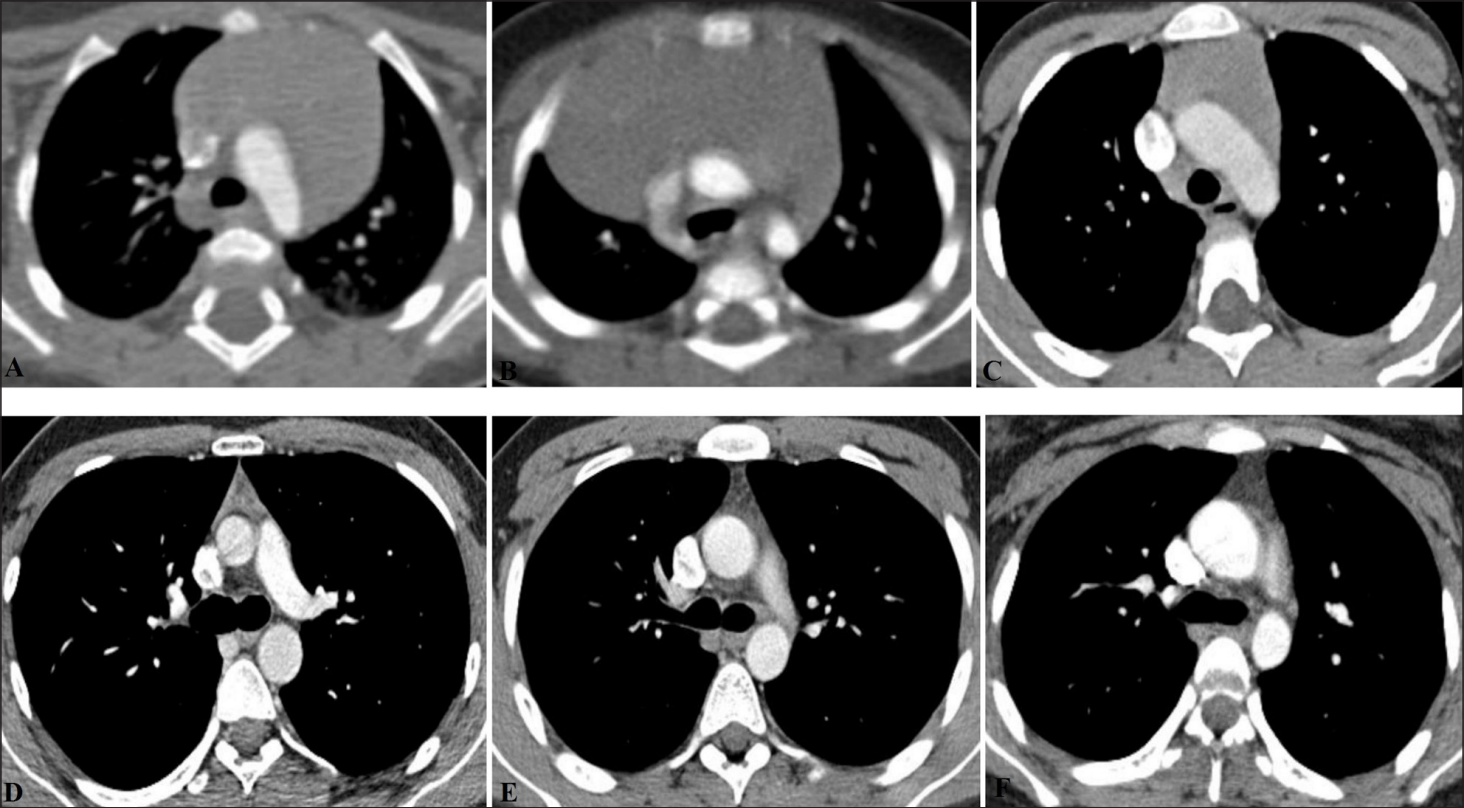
The axial thoracic contrast-enhanced computed tomography images present the morphologic features of the thymus in children. (A) 1 year old girl; round-oval shape; biconvex margins; predominantly left-sided location; Score 3, mainly soft-tissue attenuated thymus. (B) 4 months old boy; round-oval shape; biconvex margins; predominantly right-sided location; Score 3, mainly soft-tissue attenuated thymus. (C) 5 years old boy; quadrilateral shape; mixed (right straight, left convex) margins; middle location; Score 3, mainly soft-tissue attenuated thymus. (D) 15 years old boy; triangular shape; straight margins; middle location; Score 2, nearly half fatty and half soft-tissue attenuated thymus. An example of a manually delineated free-hand region of interest (ROI) for measuring the CT attenuation value is presented. (E) 17 years old boy; triangular shape; straight margins; middle location; Score 1, mainly fatty thymus. (F) 18 years old boy; triangular shape; biconcave margins; middle location; Score 0, complete fatty thymus.
